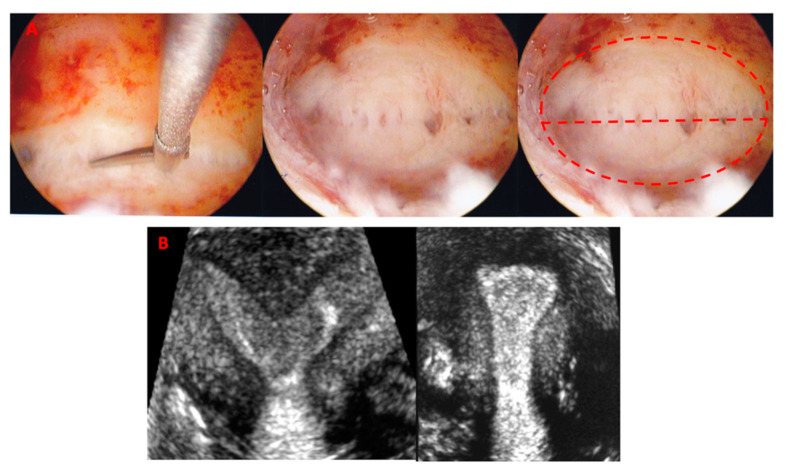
(A): surgical incision of a uterine subseptation with cold scissors (left) and resulting uterine cavity (center and right). The tubal ostia are not visualized before and soon after subseptation incision because of the wide cavity. (B): a 3-D ultrasound coronal view rendering of a subseptate uterus before and after surgical incision.
